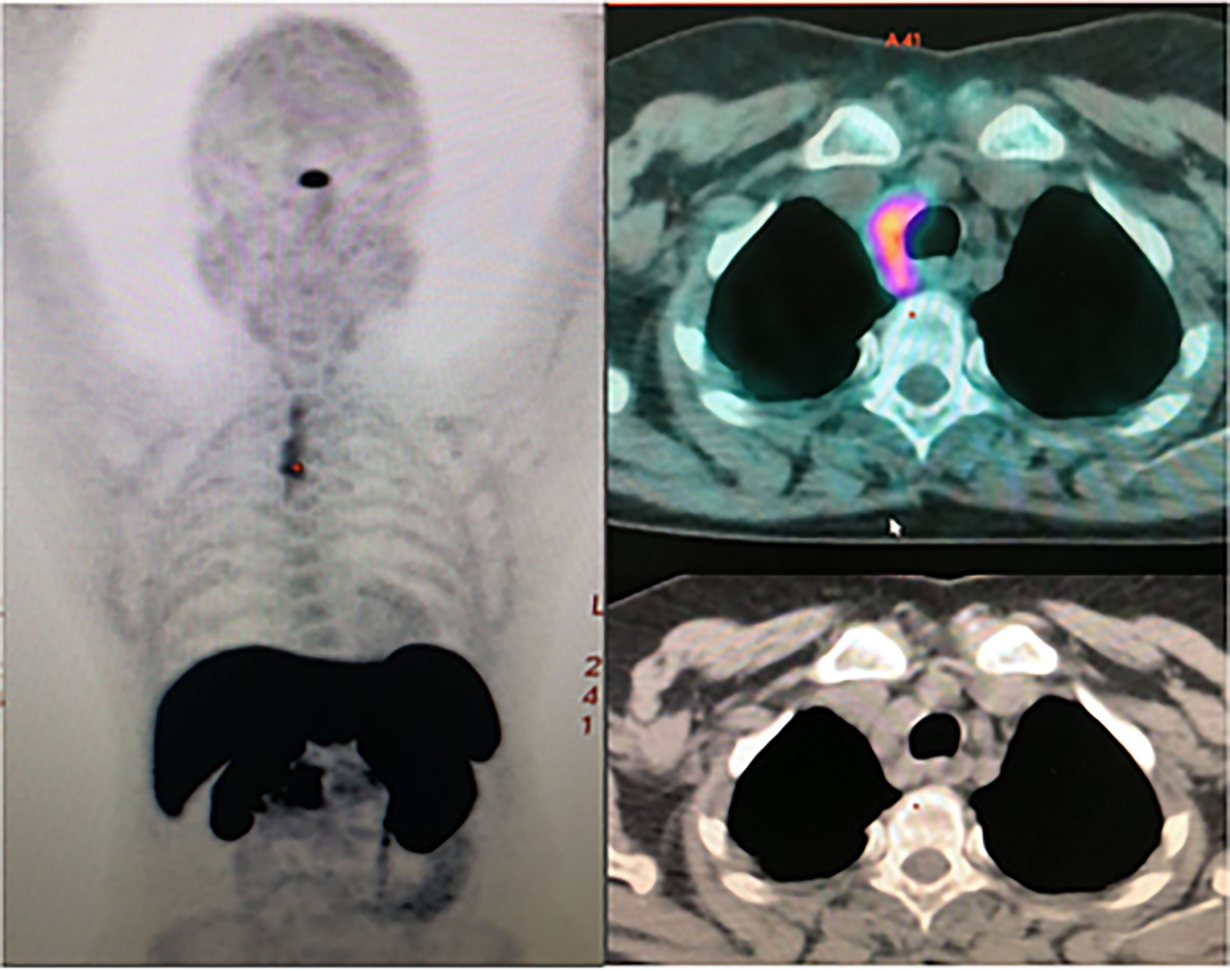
^68^Ga-DOTATATE PET/CT scan: evidence of extended uptake in the neck in then upper mediastinum.
